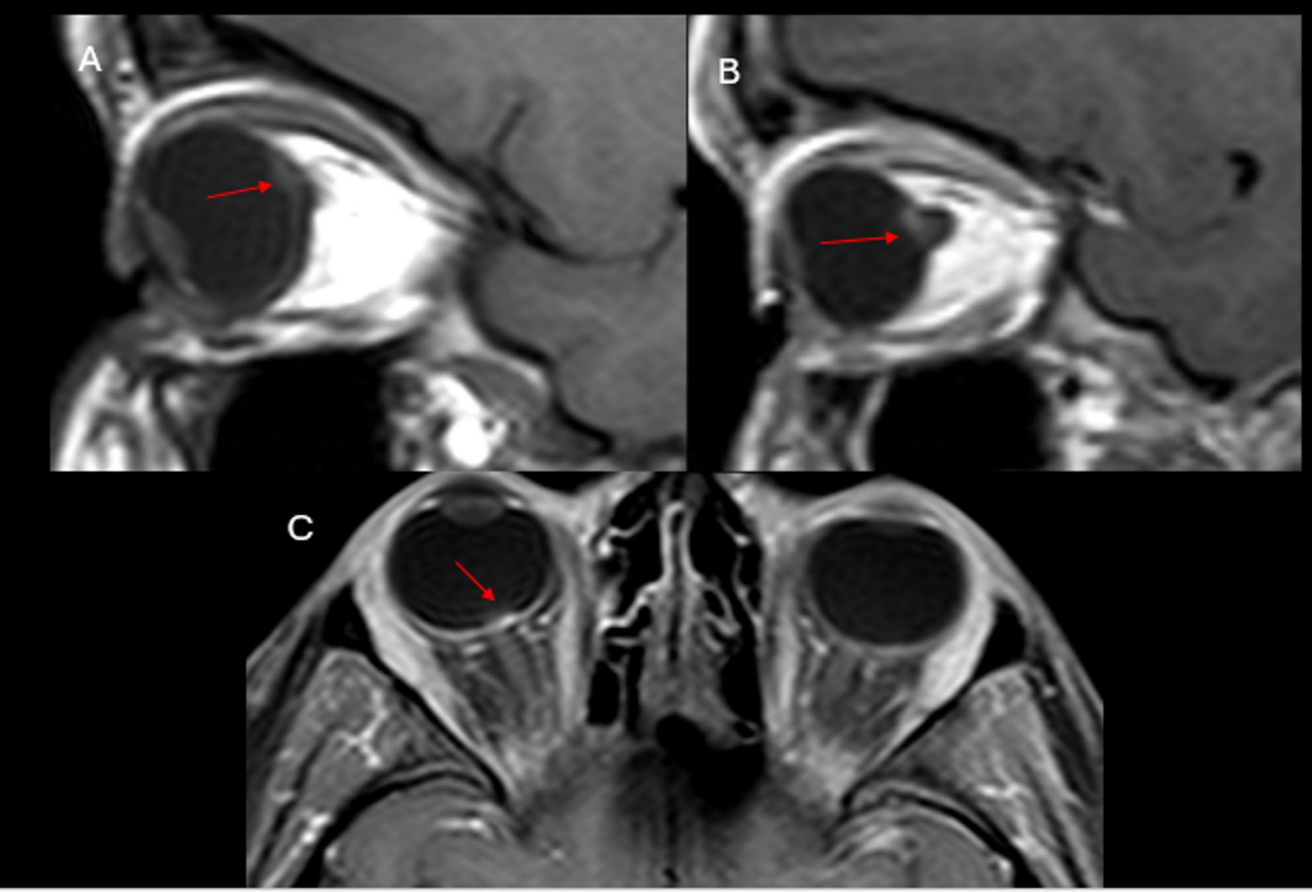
(A) Pre-contrast sagittal T1-weighted image (WI), (B) post-contrast sagittal T1WI, and (C) post-contrast axial T1-fat saturated (C) of the orbits demonstrate a small enhancing lesion in the nasal aspect of the right optic disc. It is consistent with retinal hemangioblastoma in this patient with history of Von Hippel Lindau syndrome
